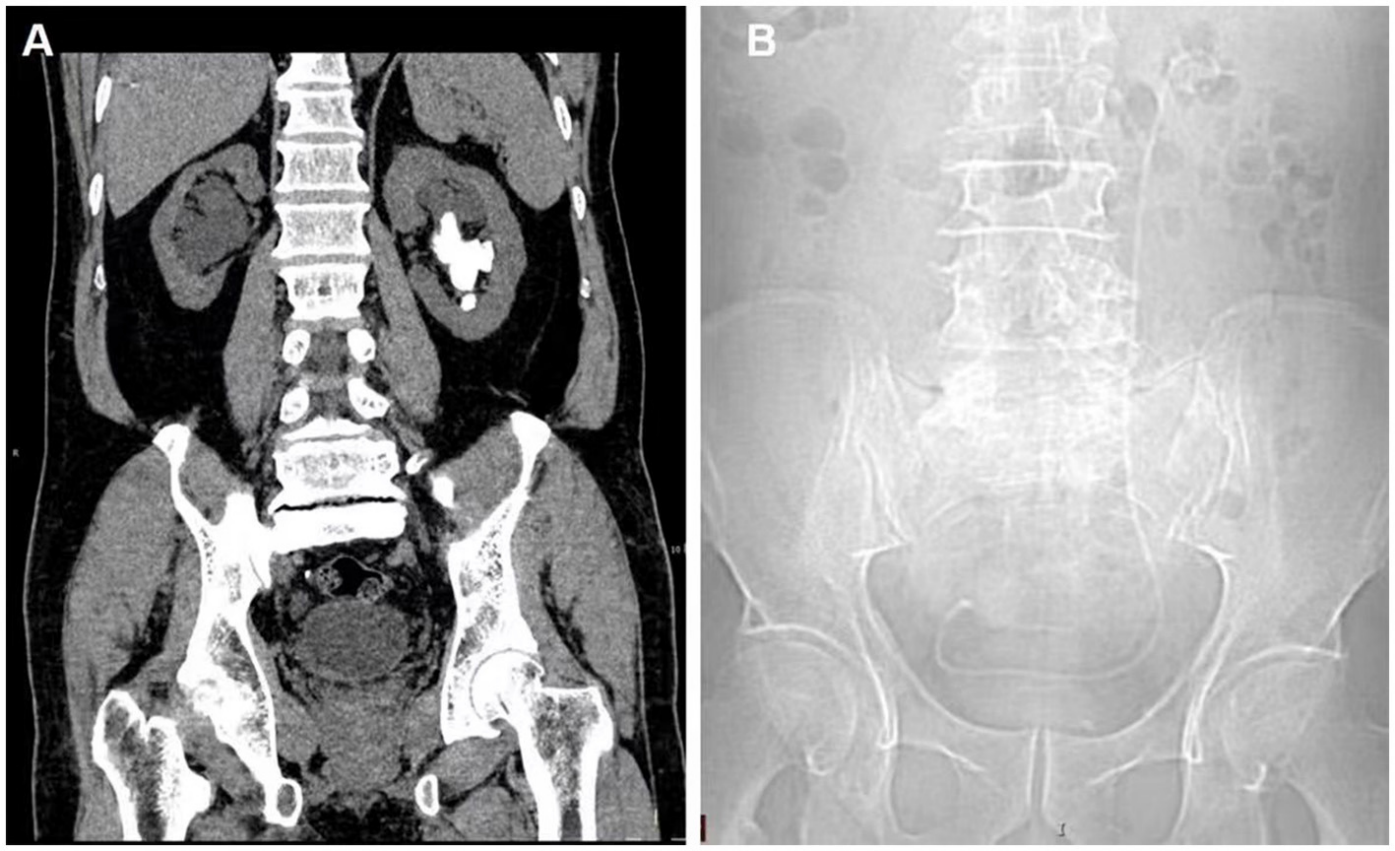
(A) CT examination before PCNL indicated left kidney stones and hydronephrosis of the right kidney. (B) Plain X-ray after PCNL showed the location of the left ureteral stent.
